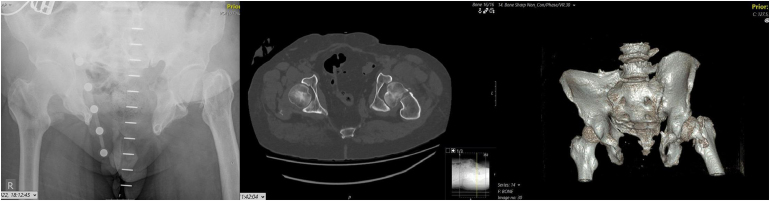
(a) Anteroposterior x-ray showing left-sided hip fracture. (b) Axial sequence of computed tomography (CT) pelvis imaging showing pubic diastasis and left-sided neck of the femur fracture. (c) Three-dimensional CT reconstruction of the pelvis
